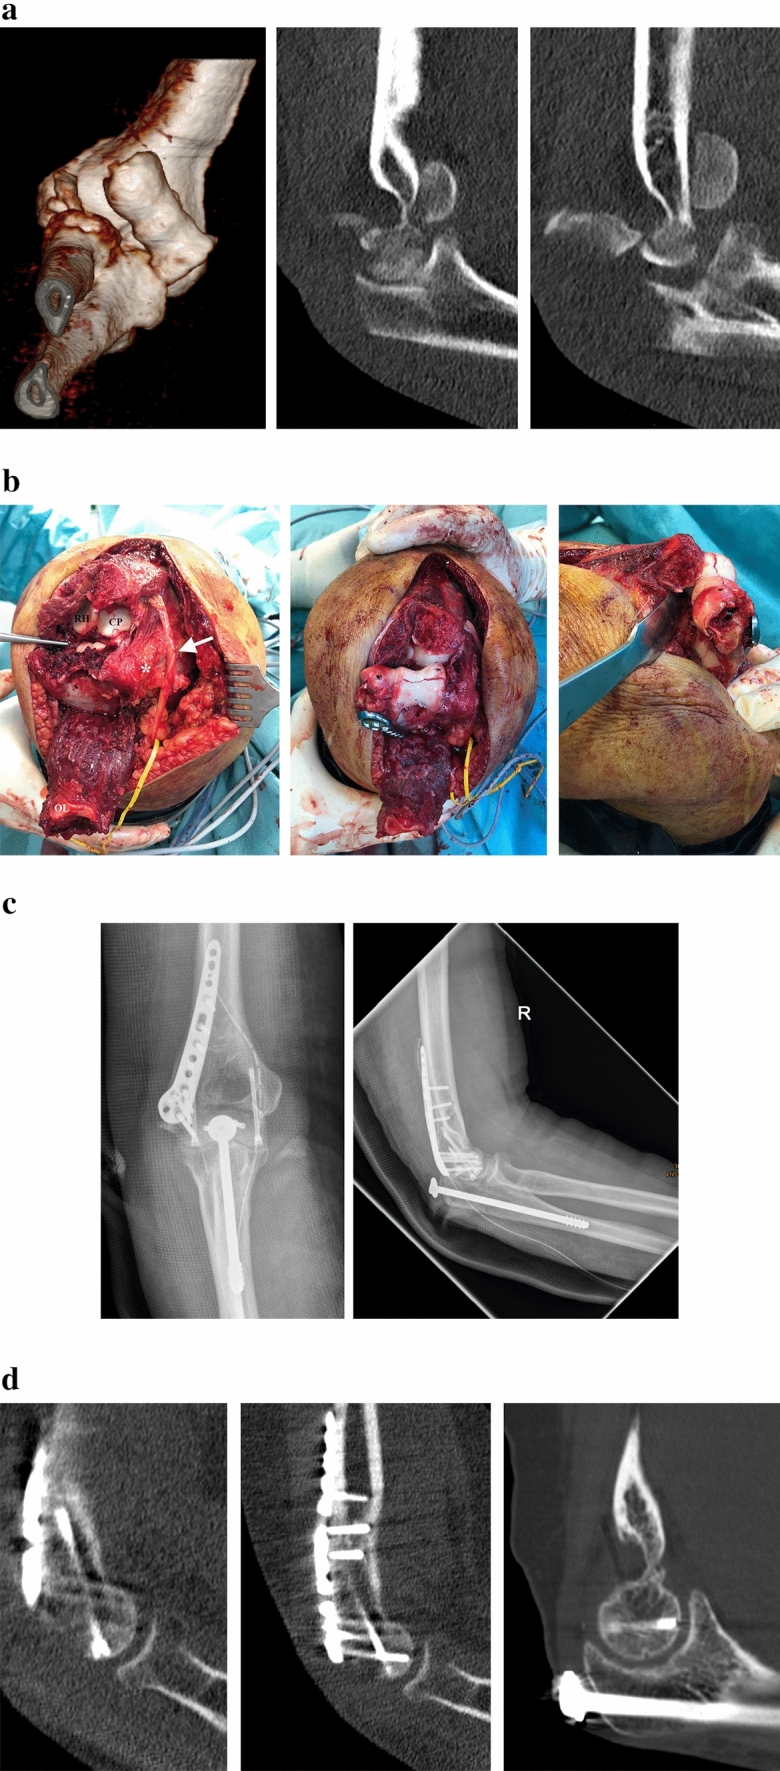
Case of a 53-year old female patient with a Dubberley type 3B fracture with concomitant radial condyle fracture of the right elbow. A Preoperative CT scan. B Intraoperative view. Using the radial condyle and olecranon fractures, a trans-fracture approach was applied to achieve ORIF while sparing the ulnar collateral ligament and the common flexor origin (*). The forceps points to the displaced articular surface. OL = Olecranon fragment; RH = Radial head; CP = Coronoid process; White arrow = Transposed ulnar nerve. C Postoperative plain radiographs. D Follow-up CT scan at one year revealing anatomic reduction and fracture union
